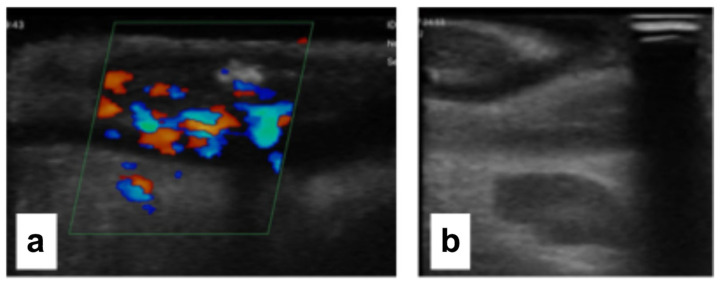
Visible transmural vascular signals are observed on color Doppler imaging (a), and a reactive lymph node (b) is discernible in the context of the mesentery in active Crohn’s disease.
